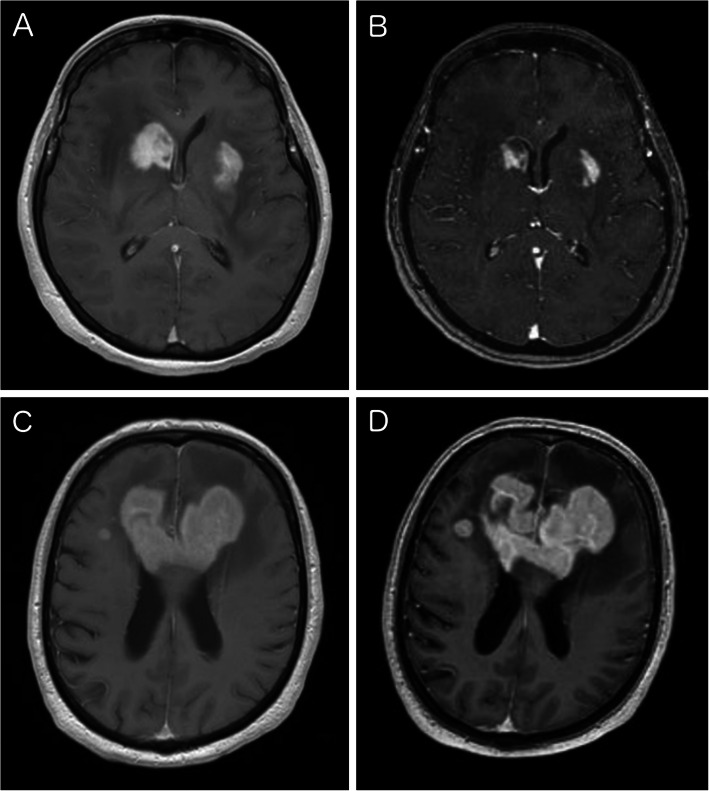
Typical MRI of PCNSL. Preoperative MRI showing multifocal contrast enhancing lesions on T1-weighted sequences (a). The patient received 12 mg dexamethasone per day for 8 days in a peripheral hospital and regression was visible on navigational MRI (b). CST was then paused 2 days prior to surgery. MRI of a patient with a large bifrontal lesion affecting the corpus callosum (c). The patient received 12 mg dexamethasone per day for 3 days following first MRI. The CST was paused immediately after transfer of the patient. Second MRI was performed after 4 days off of CST showing distinct progression (d)
